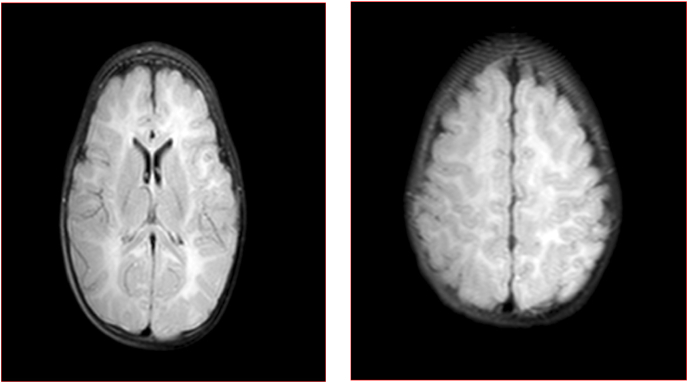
(A, B): MRI shows high signal intensity in periventricular area, up to the cortex with mild brain atrophy.
